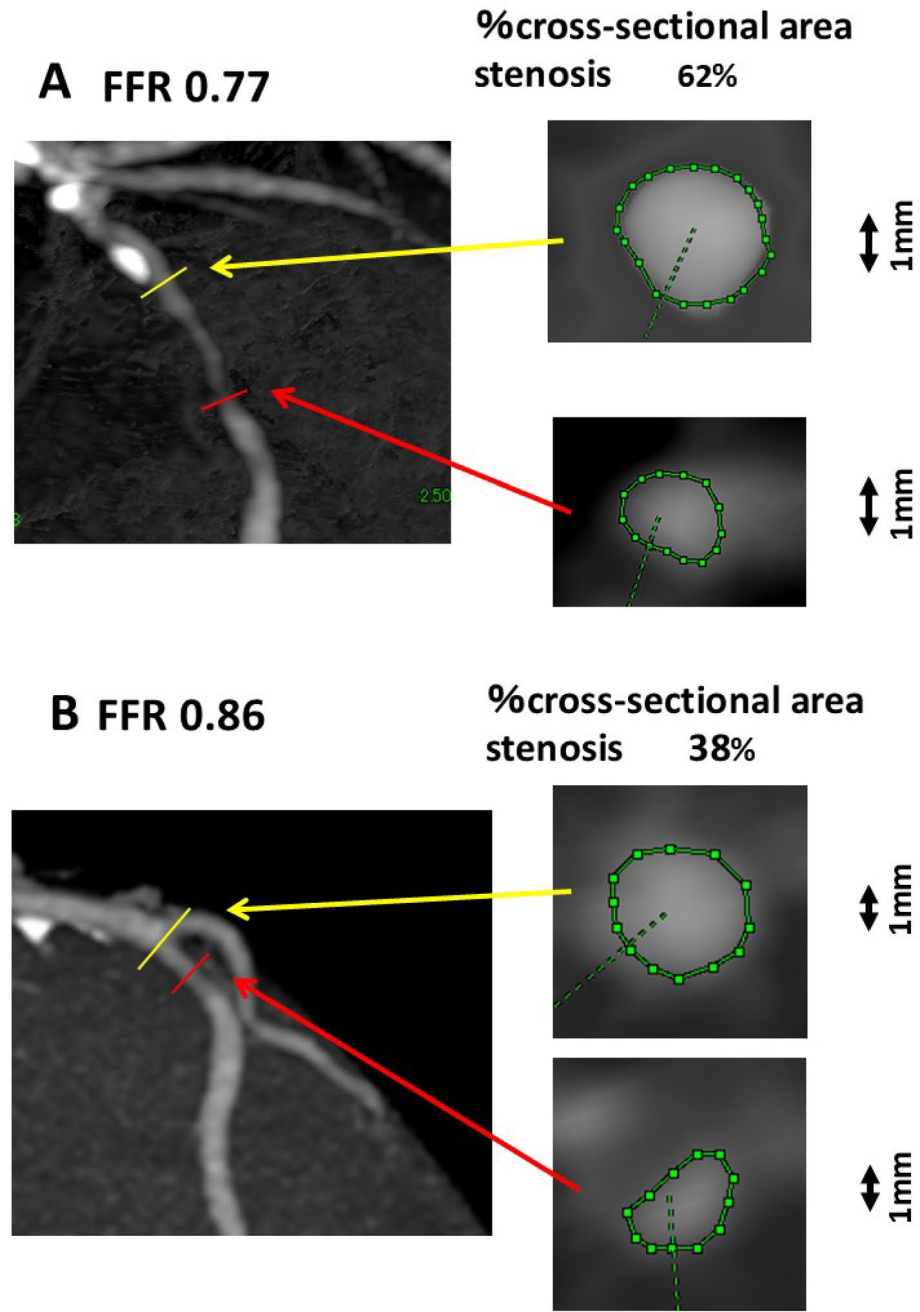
CT angiography images and cross-sectional views of a non-stenotic pre-stenosis segment (yellow arrow) and of the most stenotic segment (red arrow) are shown for two patients: one with a positive FFR (upper panel, A) and one with a negative FFR (lower panel, B). CT, computed tomography; FFR, functional flow reserve.
